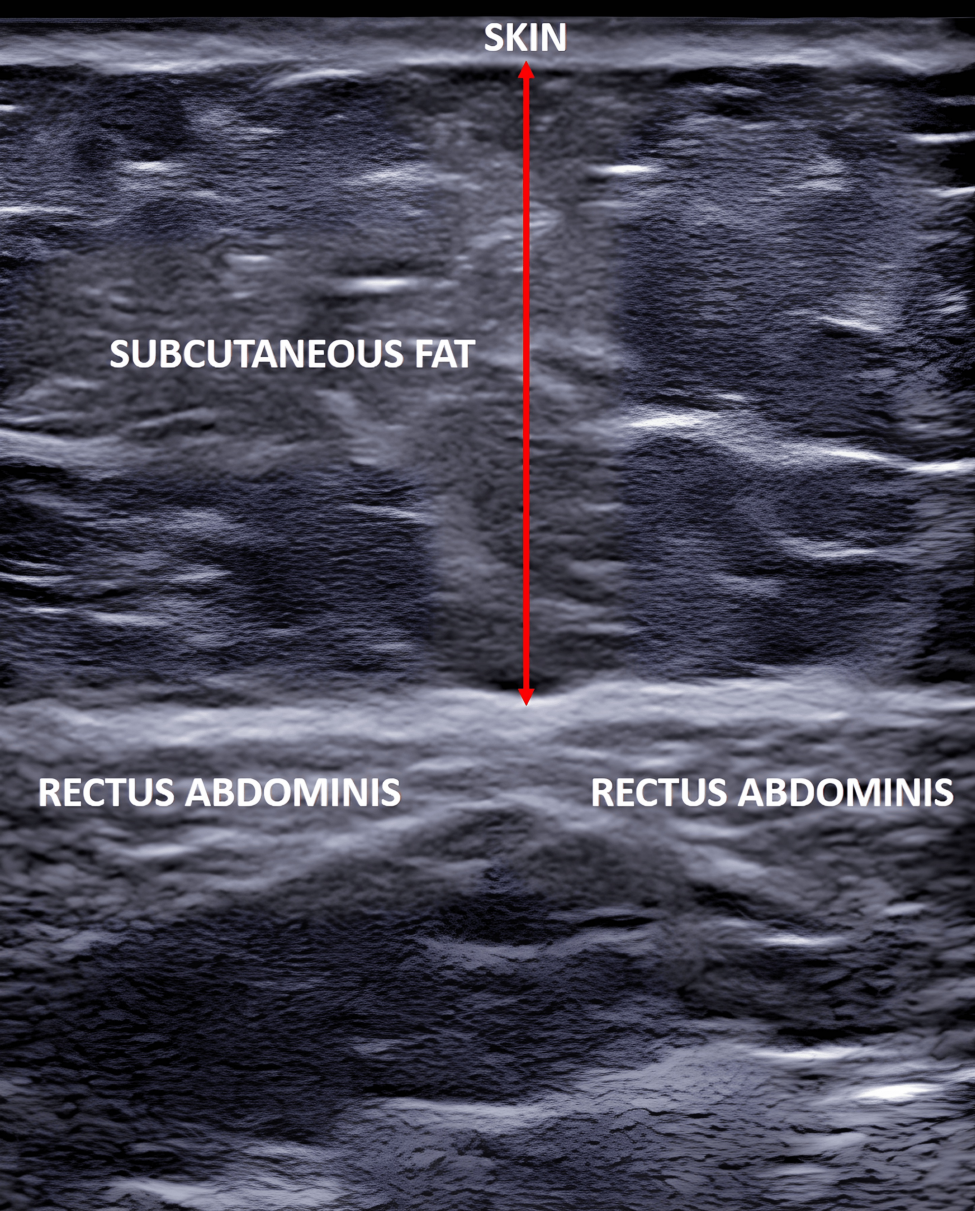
Ultrasonographic image of the subcutaneous fat of the abdomen
